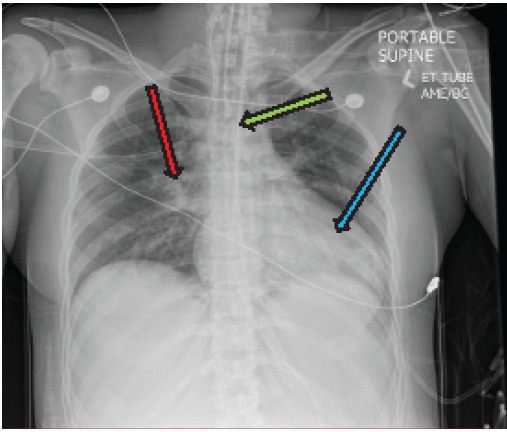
Anteroposterior chest radiograph taken in the supine position showing cardiomegaly (blue arrow) and perihilar airspace disease (red arrow), raising concern for pulmonary edema. The endotracheal tube is also seen post-intubation terminating 4.3 centimeters above the carina (green arrow).
